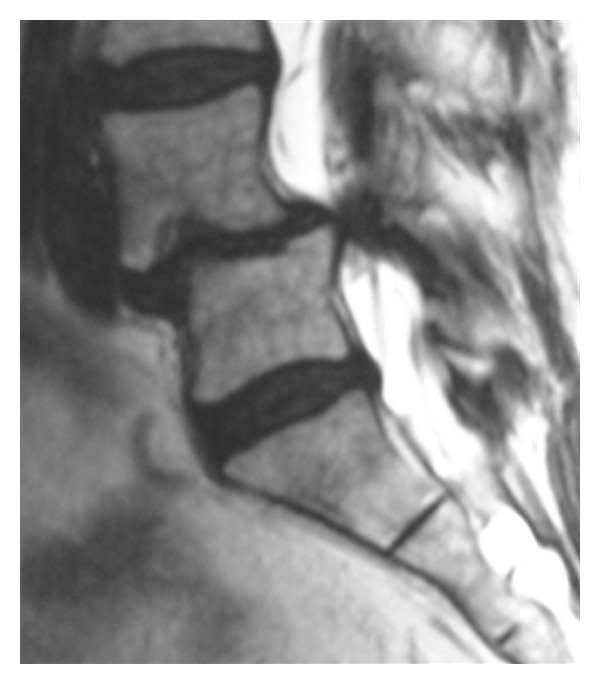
MRI scan showing spinal stenosis and spondylolisthesis.
